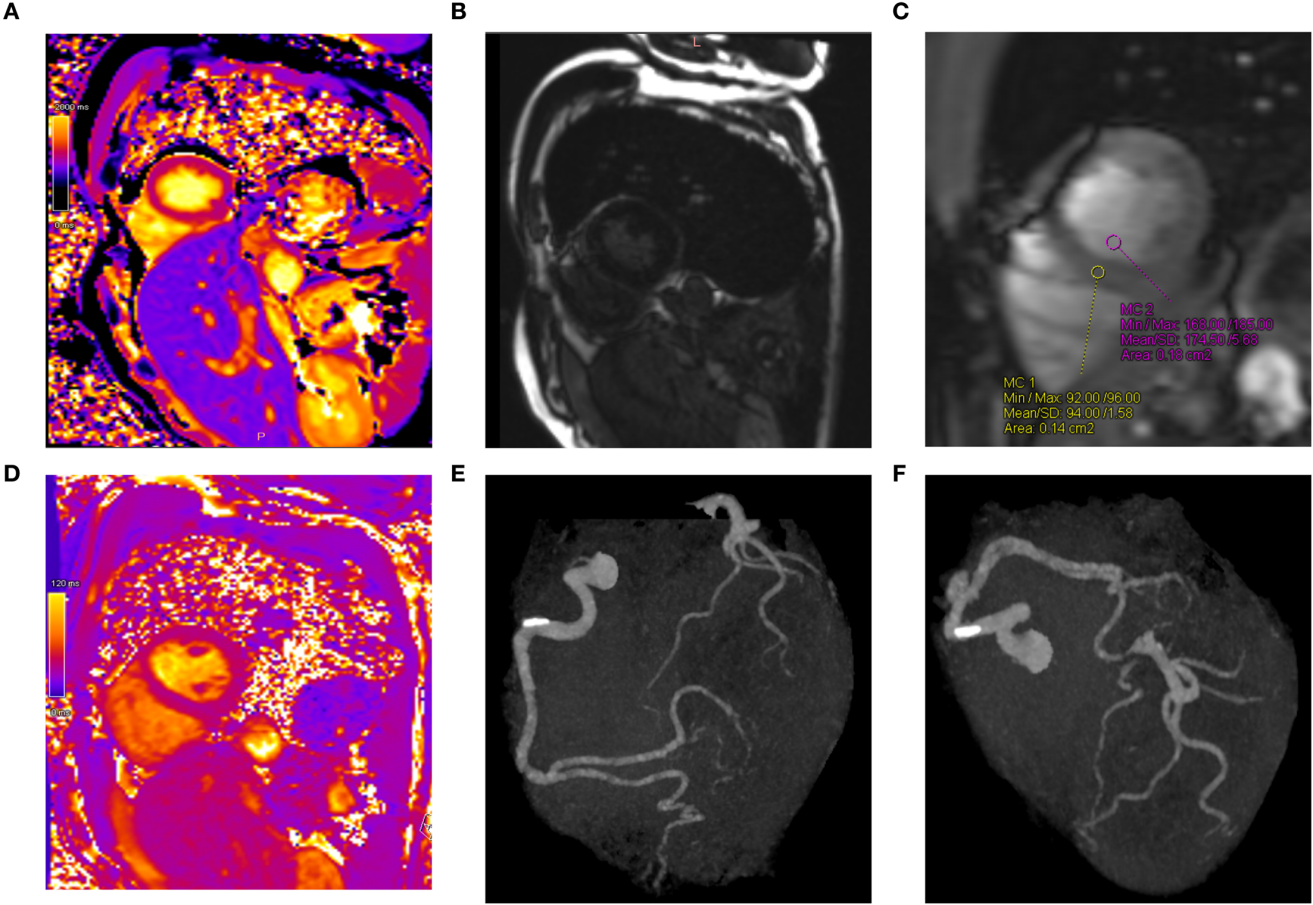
Detection of myocardial injury using cardiac imaging. (A) T1 mapping showed no clear elevation of native T1 values. (B) Delayed enhancement imaging revealed no areas of hyperenhancement. (C) Contrast-enhanced cardiac magnetic resonance imaging revealed areas of T1 shortening in the myocardium, indicative of drug-induced myocardial injury. (D) T2 mapping showed no evidence of myocardial edema. (E, F) No significant stenosis of the coronary arteries was observed on contrast-enhanced cardiac computed tomography.
